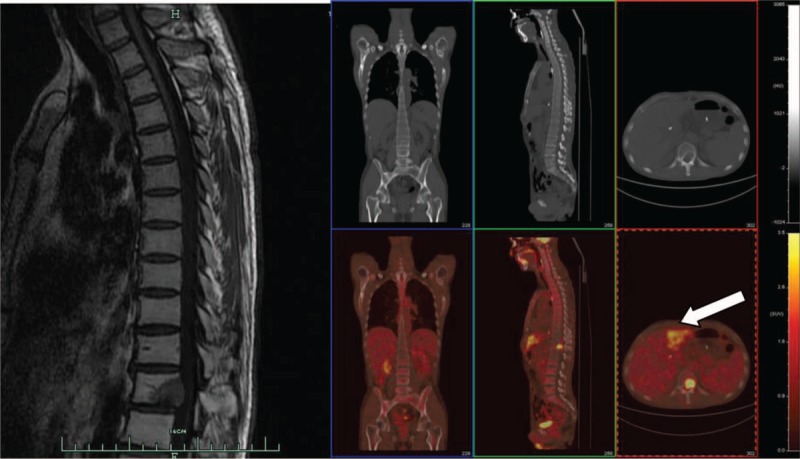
Preoperative radiographic images. Sagittal views of the T1-weighted magnetic resonance images (left) and PET (right) images. Arrows indicate the T12 metastasis and suspected recurrence of hepatocellular carcinoma in the grafted liver.
